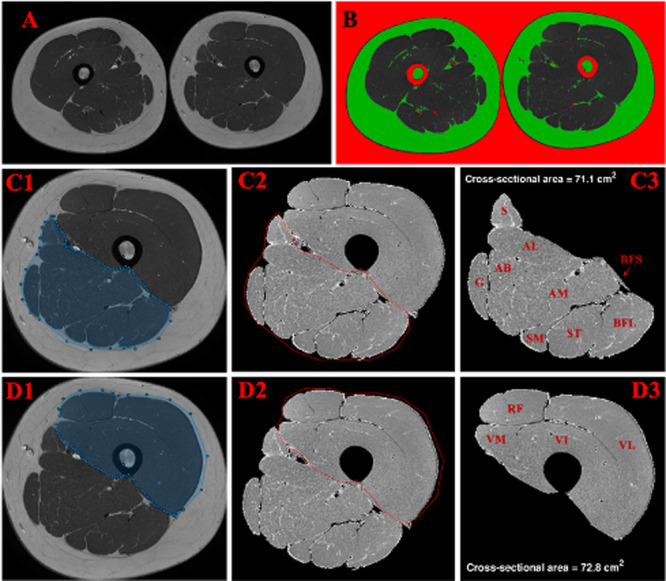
MRI thresholding and muscle segmentation using MATLAB. (A) Mid‐thigh axial T1FFE MRI acquisition. (B) MRI with thresholds applied: red = bone, green = fat, grey = muscle. Polygon ROI applied around flexor muscles (C1, C2) and extensor muscles (D1, D2). Segmentation and CSA calculation for flexor muscles (C3) and extensor muscles (D3). AB = Adductor Brevis, AL = Adductor Longus, AM = Adductor Magnus, BFL = Biceps Femoris (long head), BFS = Biceps Femoris (short head), G = Gracilis, RF = Rectus Femoris, S = Sartorius, SM = Semimembranosus, ST = Semitendinosus, VI = Vastus Intermedius, VL = Vastus Lateralis, VM = Vastus Medialis.
